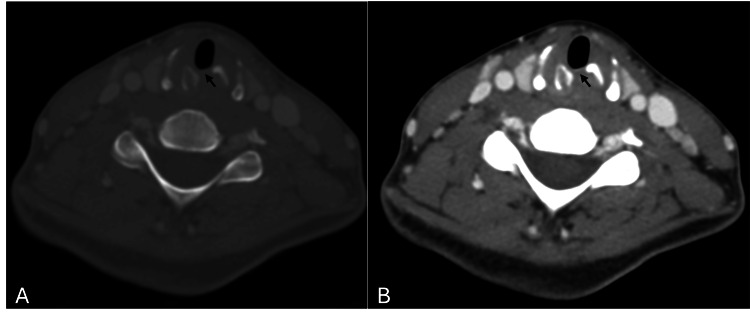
Neck CT two months after discharge (A, B) Axial computed tomography images showing complete resolution of the subglottic collection, and the known displaced fracture of the cricoid with sclerosis of the cricoid cartilage on the left (arrow).
